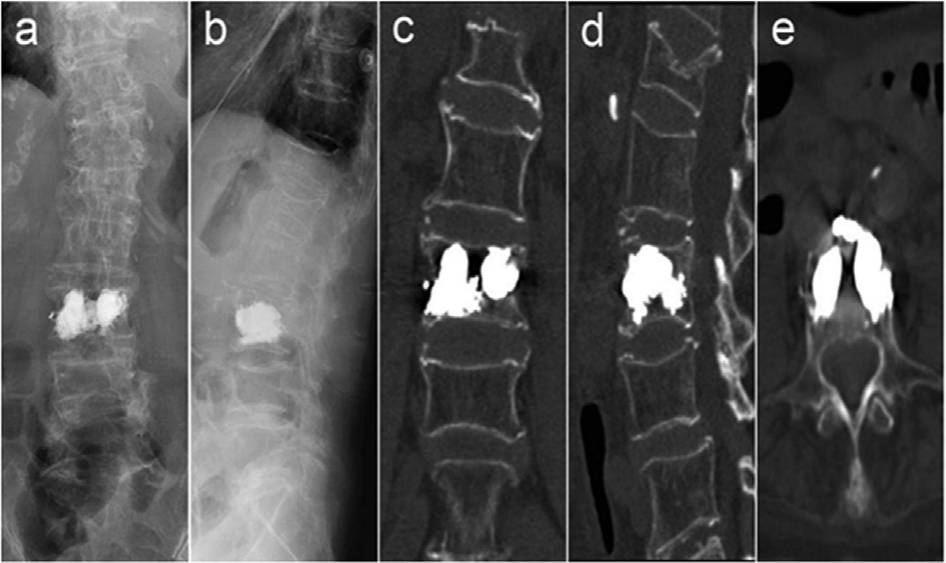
Postoperative X-ray (a, b) and crown loss, cross-sectional CT reconstruction (c, d, e) suggest that bone cement dispersion in the L3 vertebral body is good, without obvious bone cement leakage.
